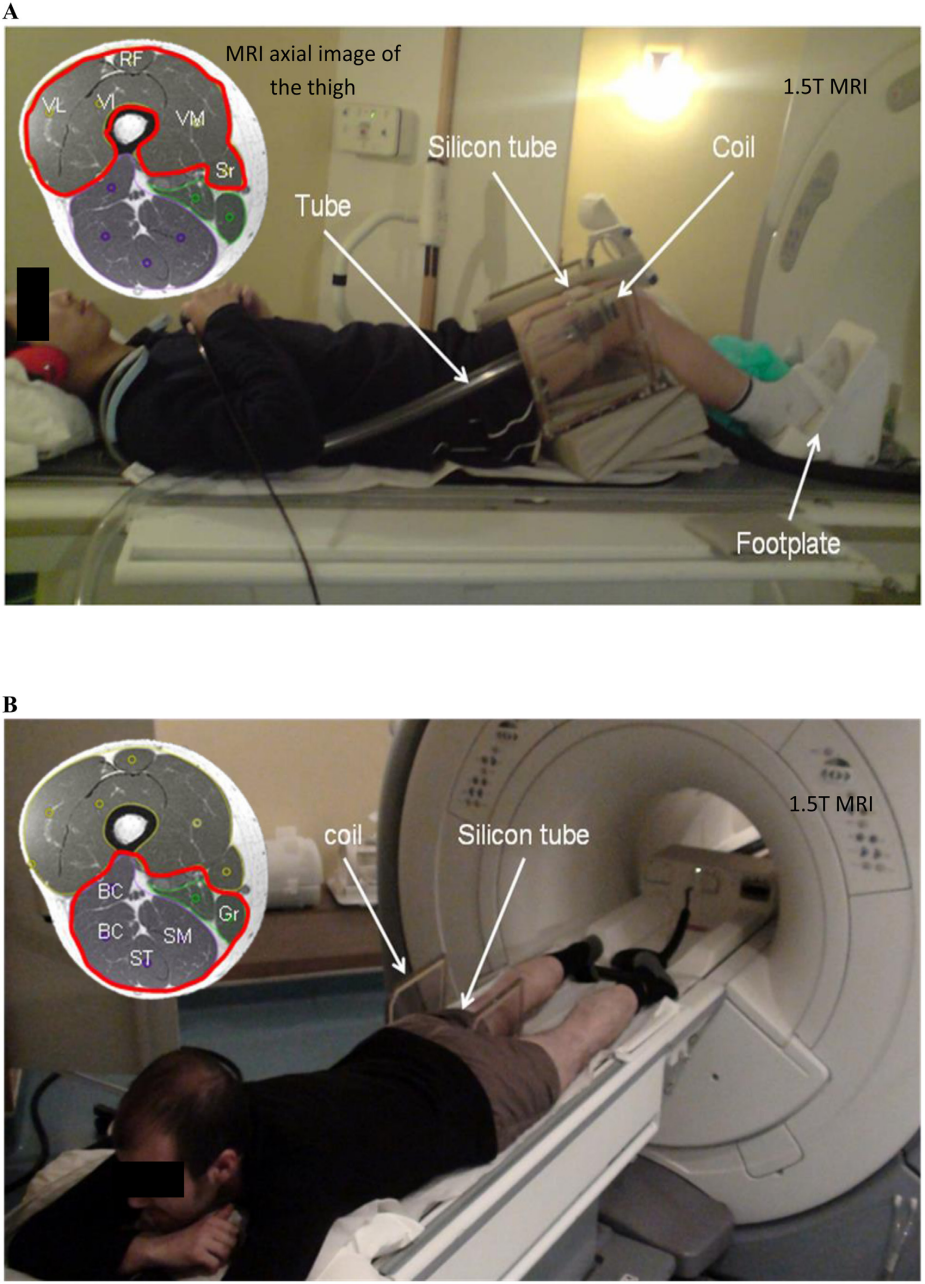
MRE setup placed inside a 1.5 T MRI machine. A: A participant laid supine on a custom-built ergometer to characterize the quadriceps (VL, RF, VI, and VM) and sartorius (Sr) muscles. B: Participant laid on his abdomen to analyze the ischio (ST, SM, BC) and gracilis (Gr) muscles. Waves were generated at 90 Hz through a pneumatic driver (silicon tube) attached around the thigh muscles, where a coil was placed. VL: vastus lateralis, RF: rectus femoris, VI: vastus intermedius, VM: vastus medialis, Sr: sartorius, ST: semitendinosus, SM: semimembranosus, BC: biceps, Gr: gracilis.
